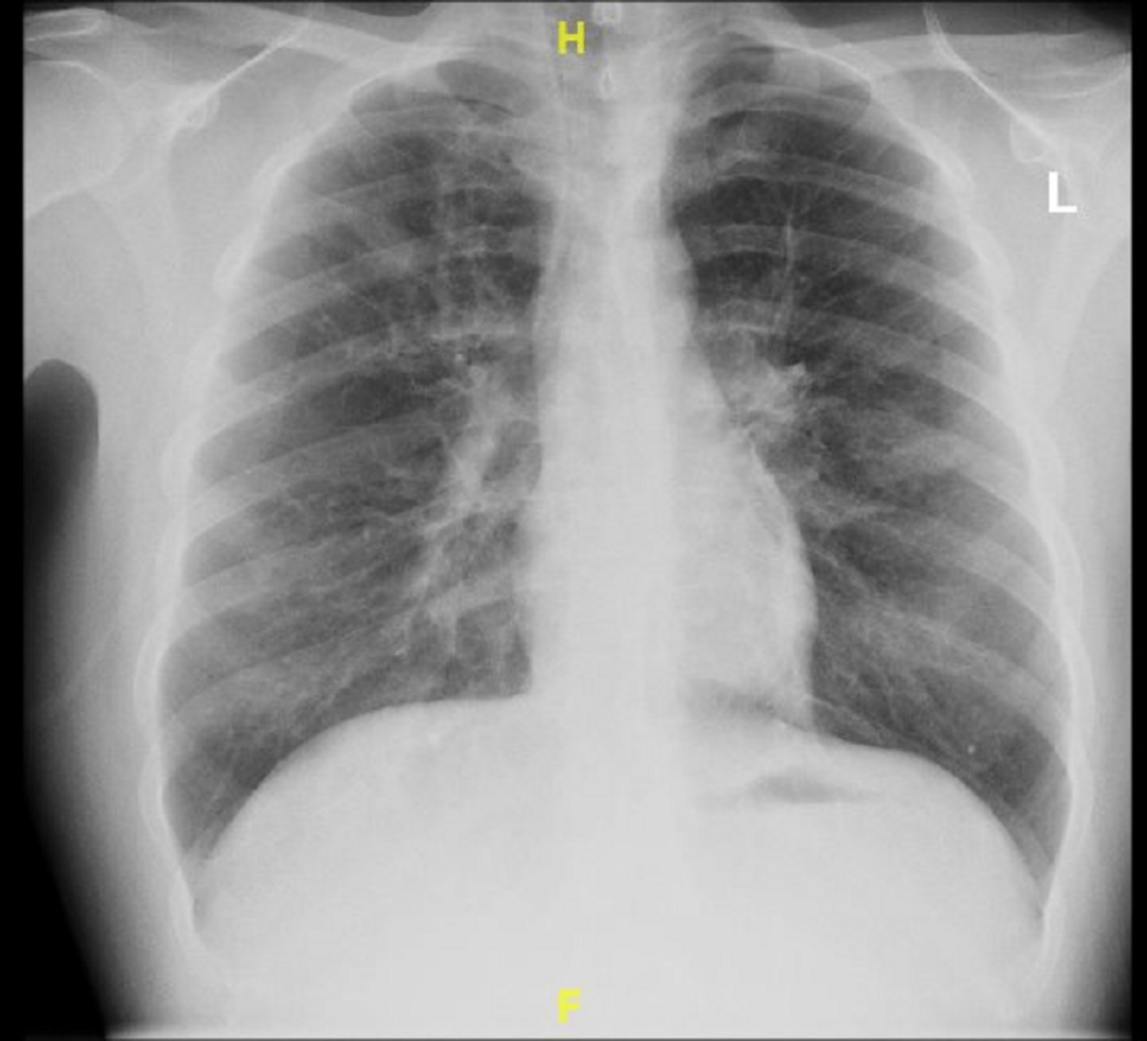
Posterioanterior Chest X-ray No acute findings
